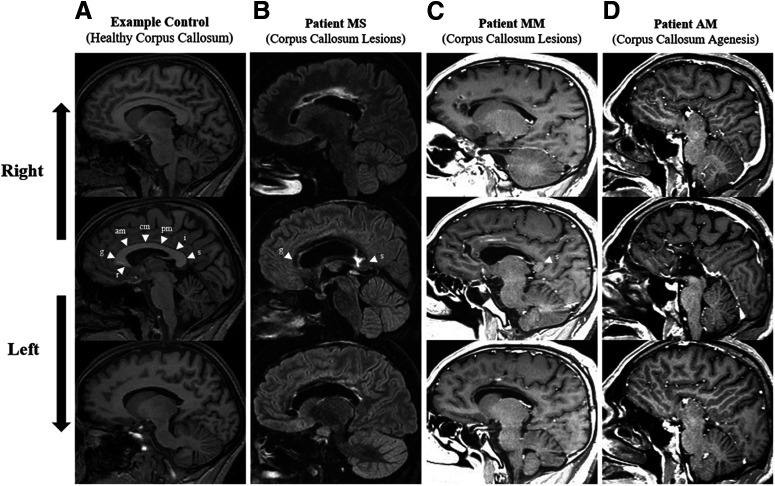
Sagittal MRI cross-section spanning from right (top row) to left (bottom row) hemisphere for (*A*) a typical control participant with complete corpus callosum (T1), (*B*) patient MS who had a brain aneurysm rupture causing lesions to the corpus callosum with only the genu (g) and splenium (s) preserved (T2-flair), (*C*) patient MM who had a stroke causing lesions to the corpus callosum with only the splenium (s) preserved (T1), and (*D*) patient AM with absent corpus callosum since birth (complete callosal agenesis; T1). Corpus callosum regions marked by white arrows are labeled on the middle row images, based on Witelson (1989), as: rostrum (r), genu (g), anterior midbody (am), central midbody (cm), posterior midbody (pm), isthmus (i), and splenium (s).
